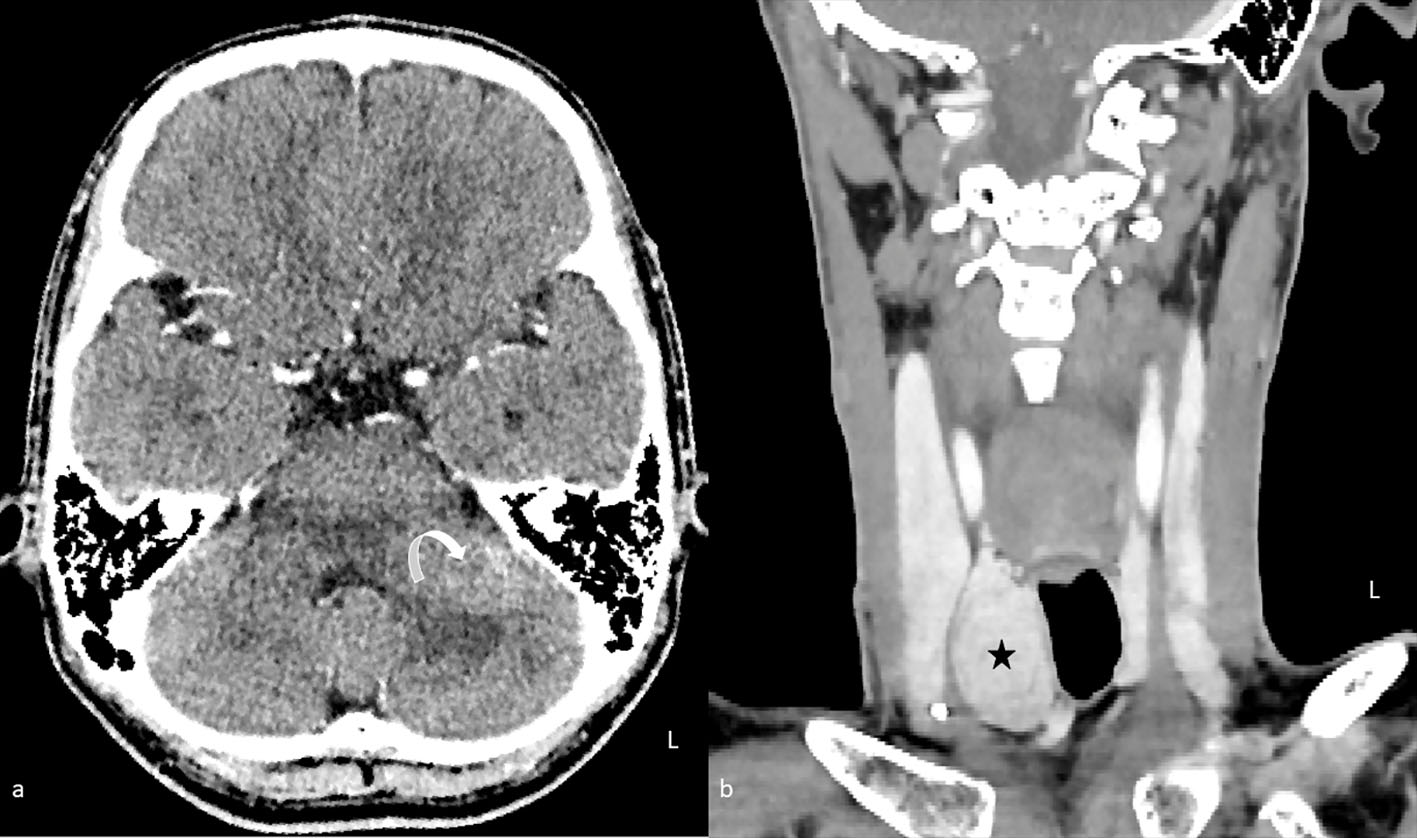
Contrast-enhanced computed tomography of the brain (A) in axial plane and neck (B) in coronal plane. An enhancing lesion in the left middle cerebellar peduncle is causing mass effect with mild effacement of the fourth ventricle (curved arrow). A right thyroid nodule is also seen synchronously (star).
